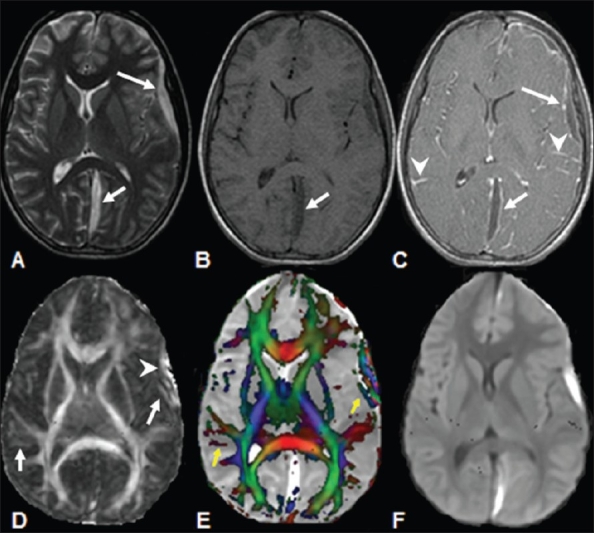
A 14-year-old patient with meningitis with an associated subdural collection of pus in the frontotemporal region and interhemispheric fissure. The T2W axial image (A) shows hyperintensity in the frontotemporal region and interhemispheric fissure (arrows), which appears hypointense (arrows) on the T1W image (B) and shows wall enhancement (arrows) on the post-contrast T1W (C) image. Meningeal enhancement (arrowheads), consistent with meningitis, is also seen on this image. The FA map (D) shows increased FA values (arrowhead) in the subdural collection as well as in the cortical grey matter region (arrows), which enhances on the post-contrast T1W image. The abnormality (yellow arrow) is more clearly visible on the color-coded FA map (E) modulated by the principal eigenvector. The DWI image (F) shows areas of restricted diffusivity in the subdural collection
